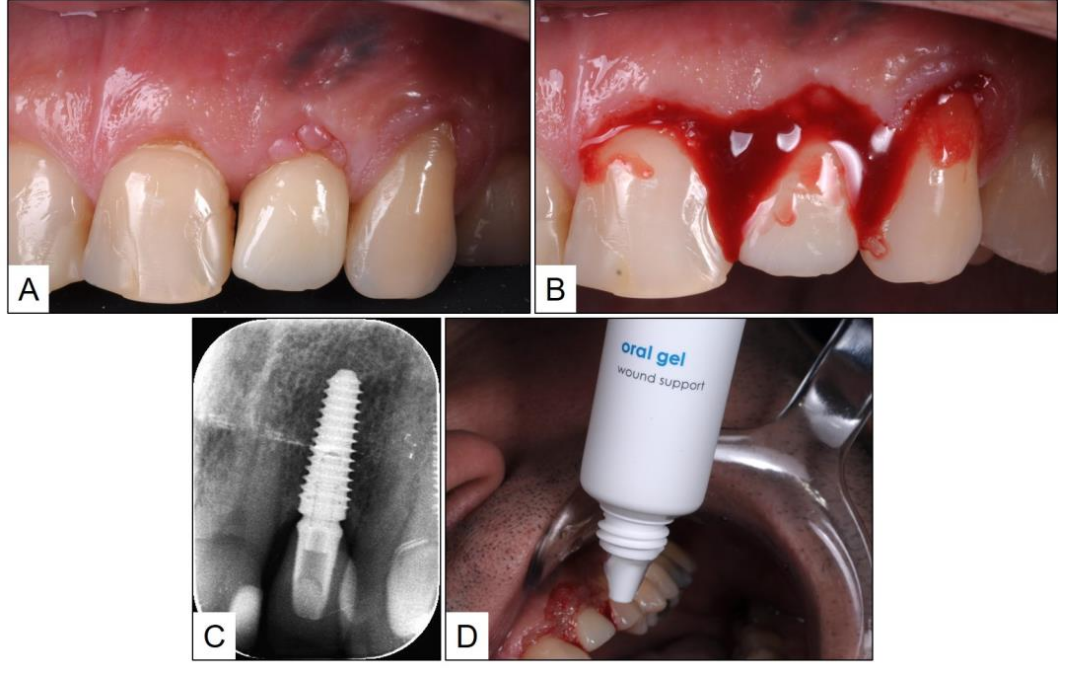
After two years of crown delivery: (A) Patient presented with marginal redness of the peri-implant mucosa in #22 area; (B) Clinically there was severe bleeding on probing; (C) Periapical radiographs revealed marginal peri-implant bone level at the same height as implant shoulder; and (D) Topical application of blue^®^m oral as part of treatment using TOOTh protocol.
